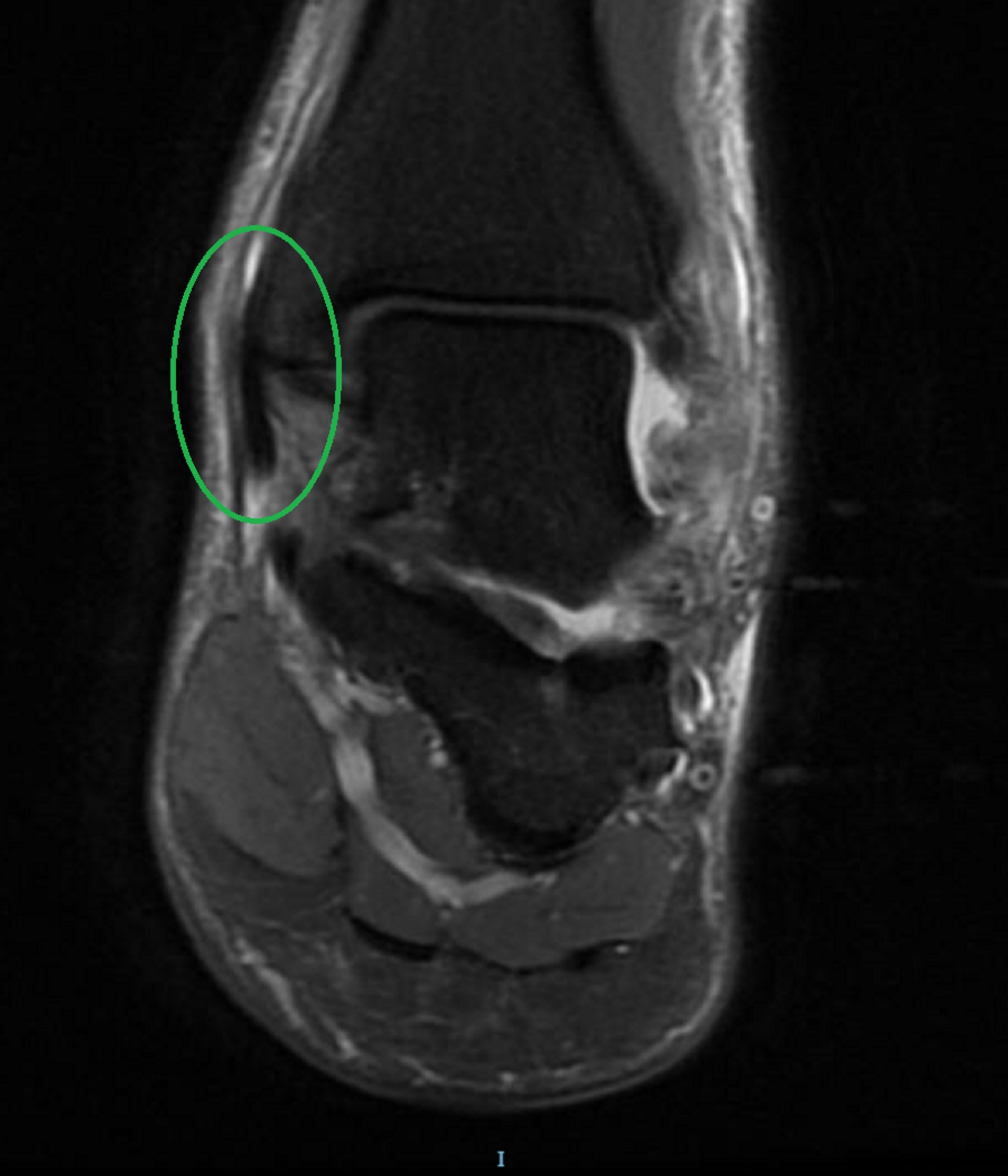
T2-weighted MRI (coronal plane) demonstrating an anterior subluxation of the posterior tibialis tendon over the medial malleolus with mild effusion (green circle), which should appear posterior to the medial malleolus.
